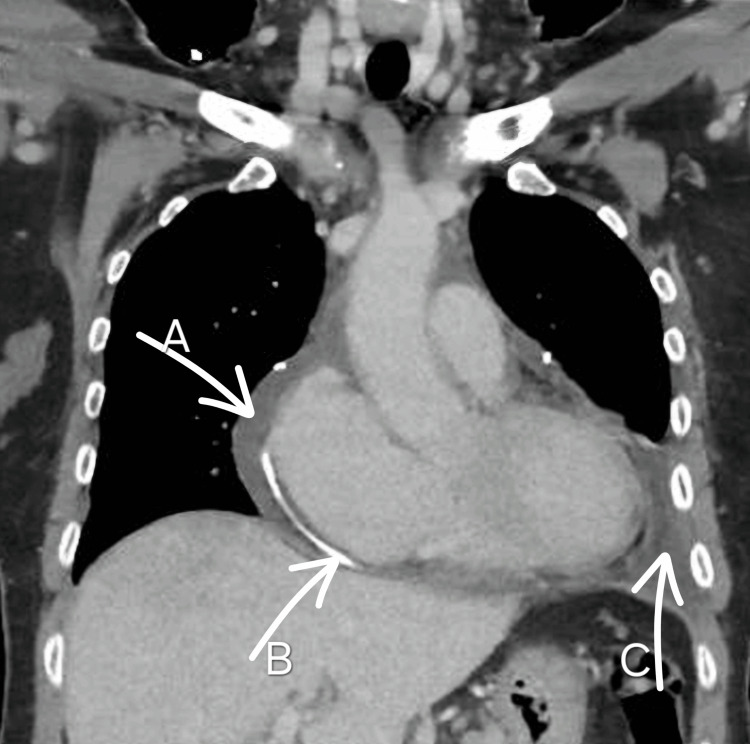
Contrast-enhanced coronal CT image of the thorax, showing a moderate pericardial effusion (arrow A) and a left-sided pleural effusion (arrow C). Notably, a pigtail catheter (arrow B) is visualized within the pericardial space, indicative of ongoing or recent pericardiocentesis for therapeutic drainage CT: computed tomography
